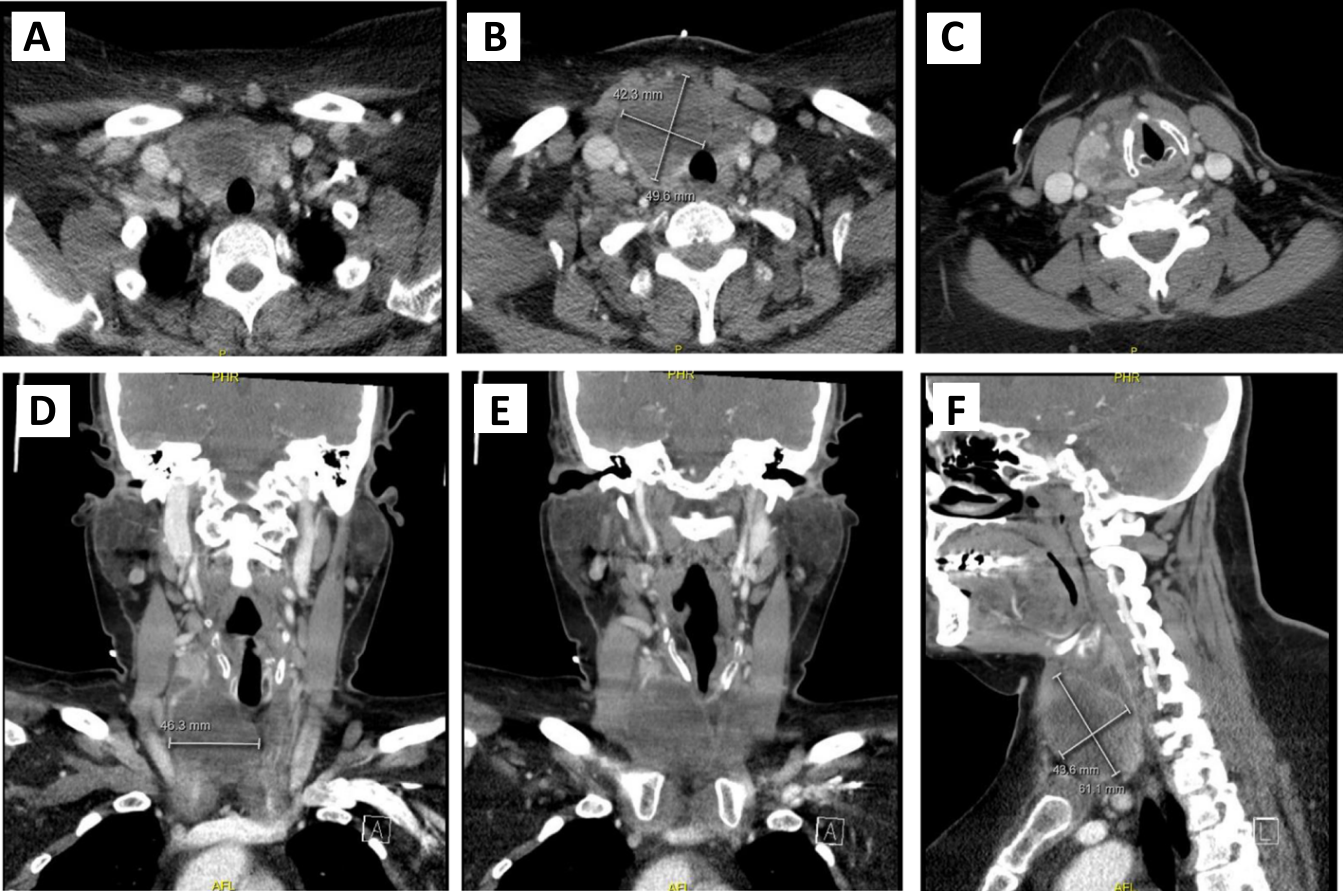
An enhanced CT scan of the neck on initial presentation. a-c showing the axial views from superior to inferior, d-f showing the coronal views from anterior to posterior and f showing the sagittal view. Images are showing a large lobulated cystic lesion with some thin septations, measuring about 6.1 × 4.4 × 4.6 cm in maximal craniocaudal, anterior-posterior, and transverse dimensions, respectively
